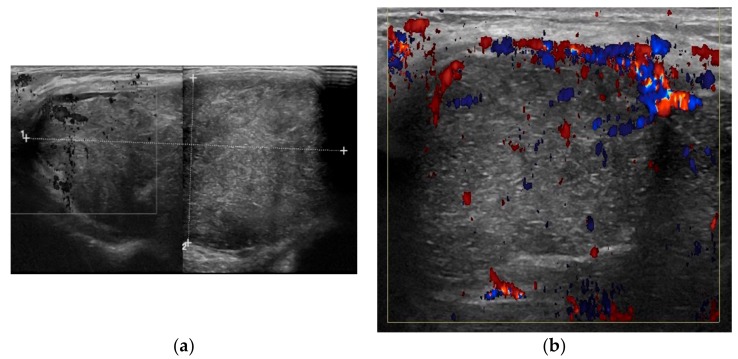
(a) Breast ultrasound (US) shows hyperechoic wall; scattered internal echoes and hypoechoic peripheral lacunae of colliquative nature; and (b) Doppler US demonstrated increased peripheral and intralesional vascularity with axillary reactive lymphadenopathy.
